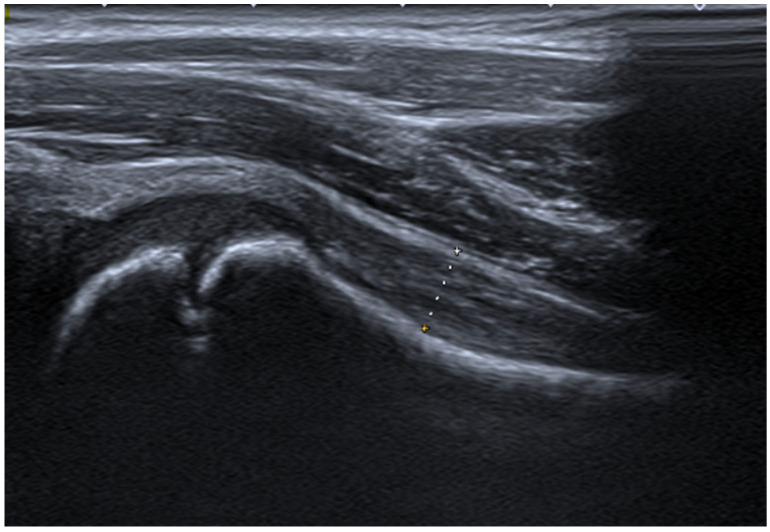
Sagittal imaging of the hip joint synovial cavity thickness in a 4-year-old patient using an anterior longitudinal sonographic scan.
